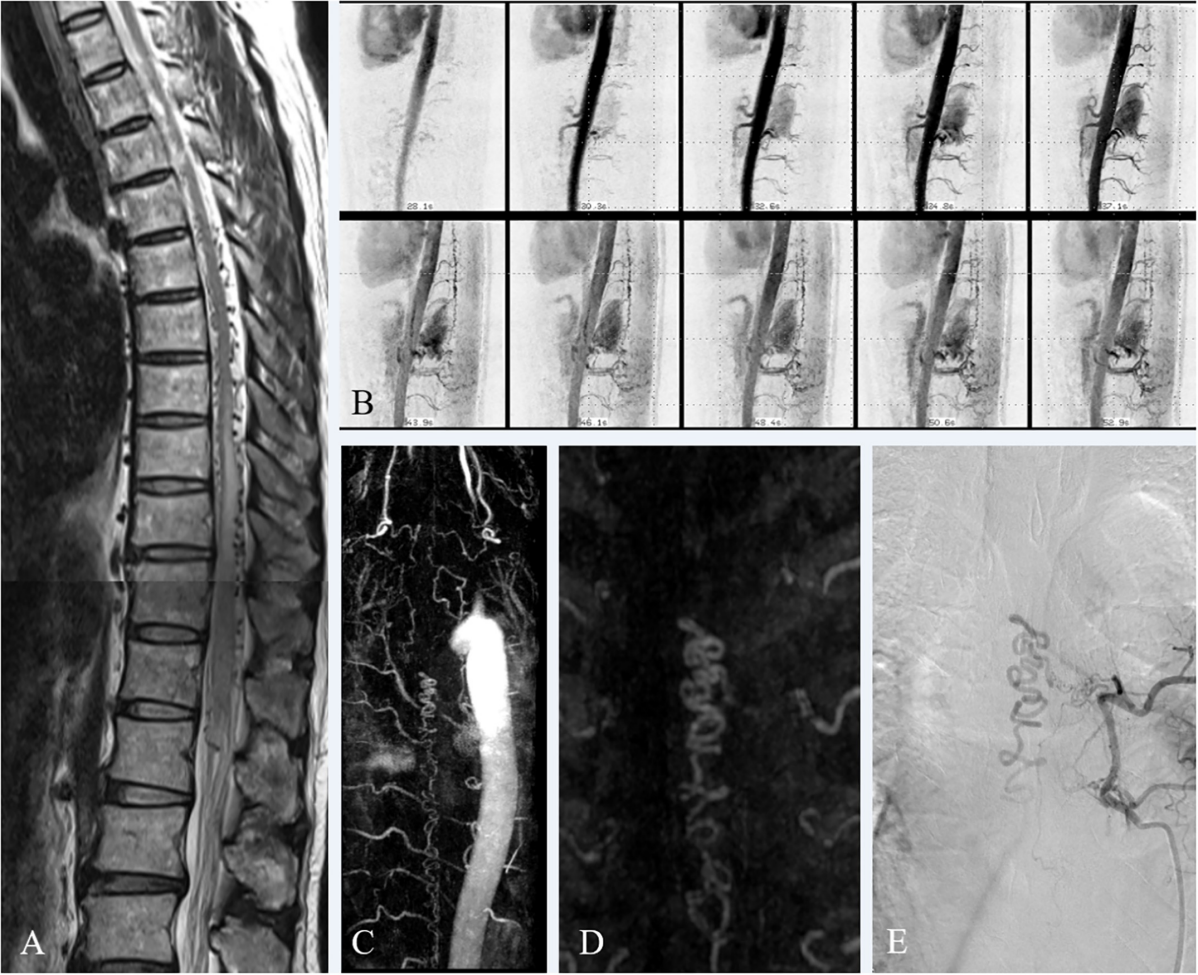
A 53-year-old man with spinal dural arteriovenous fistula (DAVF) (patient 1). T2-weighted sagittal image (A) revealing tortuously dilated perimedullary veins in the thoracic and lumbar spinal canal. Serial maximum intensity projection (MIP) images of low-dose time-resolved contrast-enhanced magnetic resonance angiography (CE-MRA) (B) revealing sequential visualization of dilated perimedullary veins from the top of the field-of-view. MIP images of the entire field of view (C) and coronal segmental MIP image (D) of three-dimensional single-phase high-resolution CE-MRA revealed fistula at the left T6 level. Spinal digital subtraction angiography (E) confirmed spinal DAVF with shunt located at the matched level.
